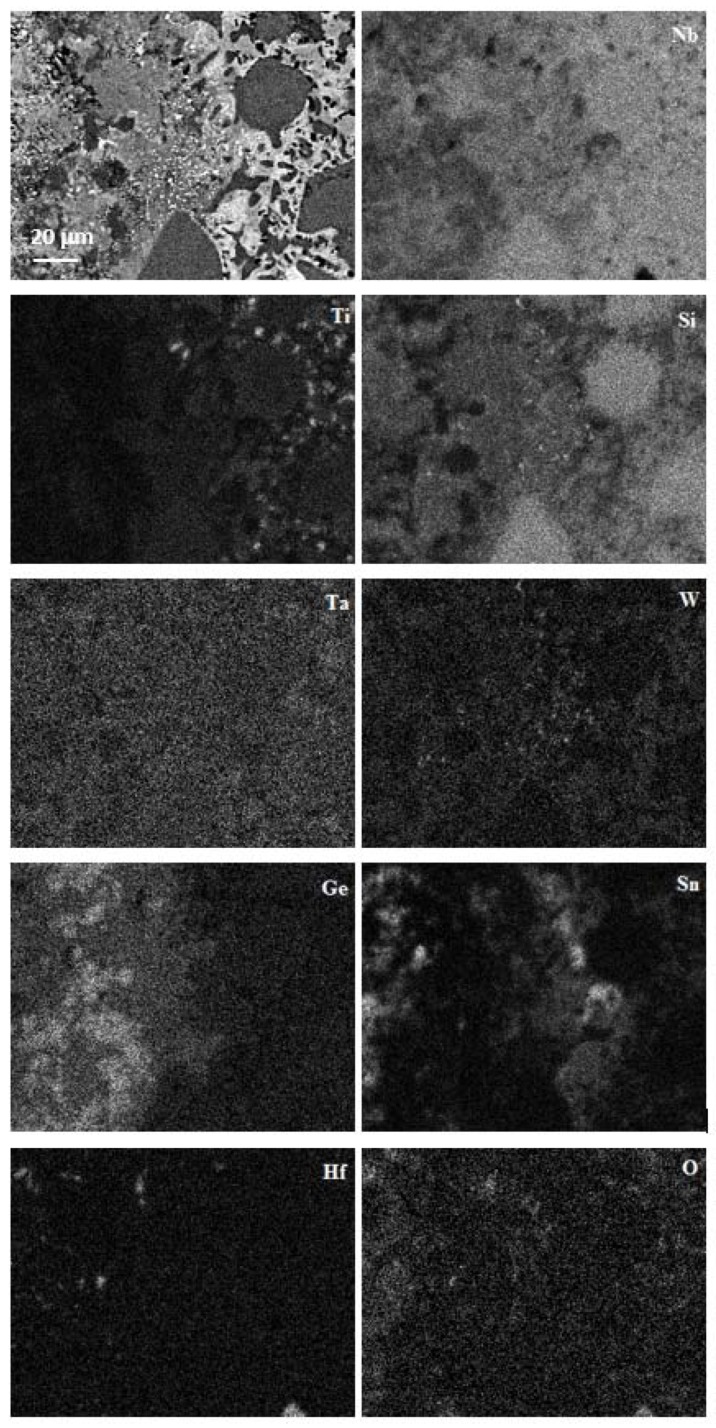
BSE image and X-ray maps of the rectangular region in Figure 8d.
